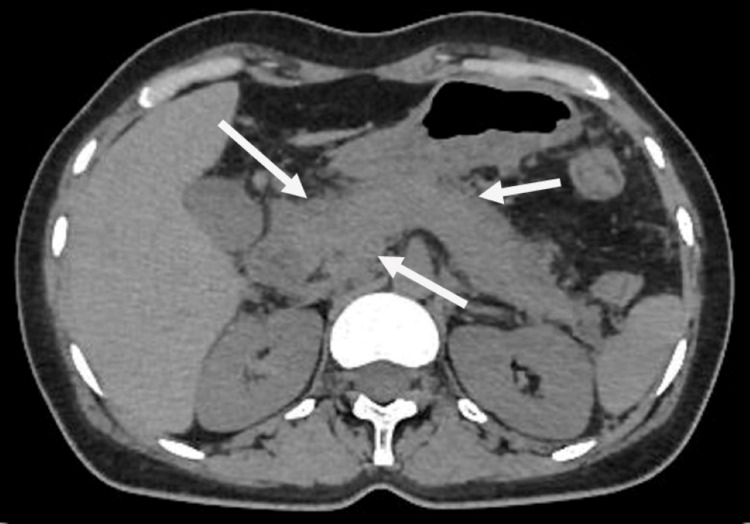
Abdominal CT revealing diffuse enlargement of pancreas with imprecise borders.
